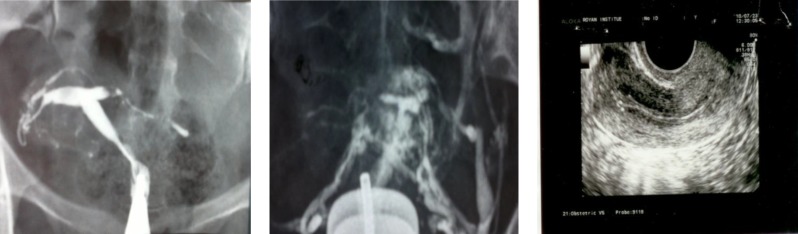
Case 3. A) HSG 4 years ago, bilateral tubal obstruction with golf-club appearance, B) Recent HSG revealed T shaped uterus, Venous and lymphatic intravasation. C) Uterine ultrasound, tiny foci of calcification within the endometrium which shows some degree of adhesion
